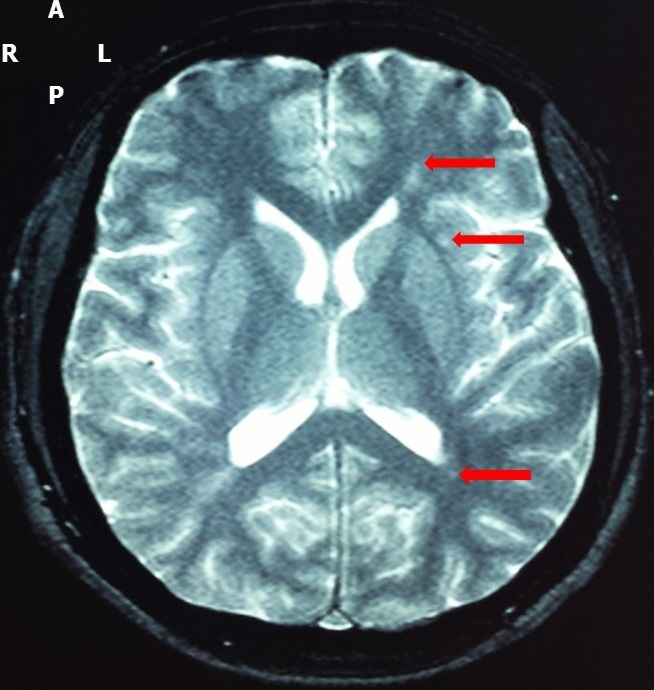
Axial T2-weighted brain MRI showing (in red arrows) hypersignal lesions located in the left semi-oval center and periventricular area: A = anterior; P = posterior; L = left; R = right
